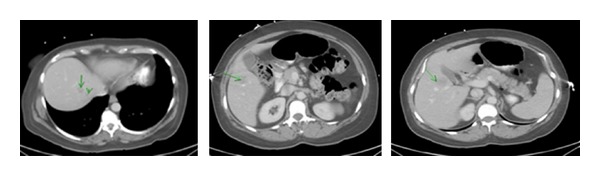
Computed tomography of the abdomen showing multiple metastatic lesions in various segments of the liver.
